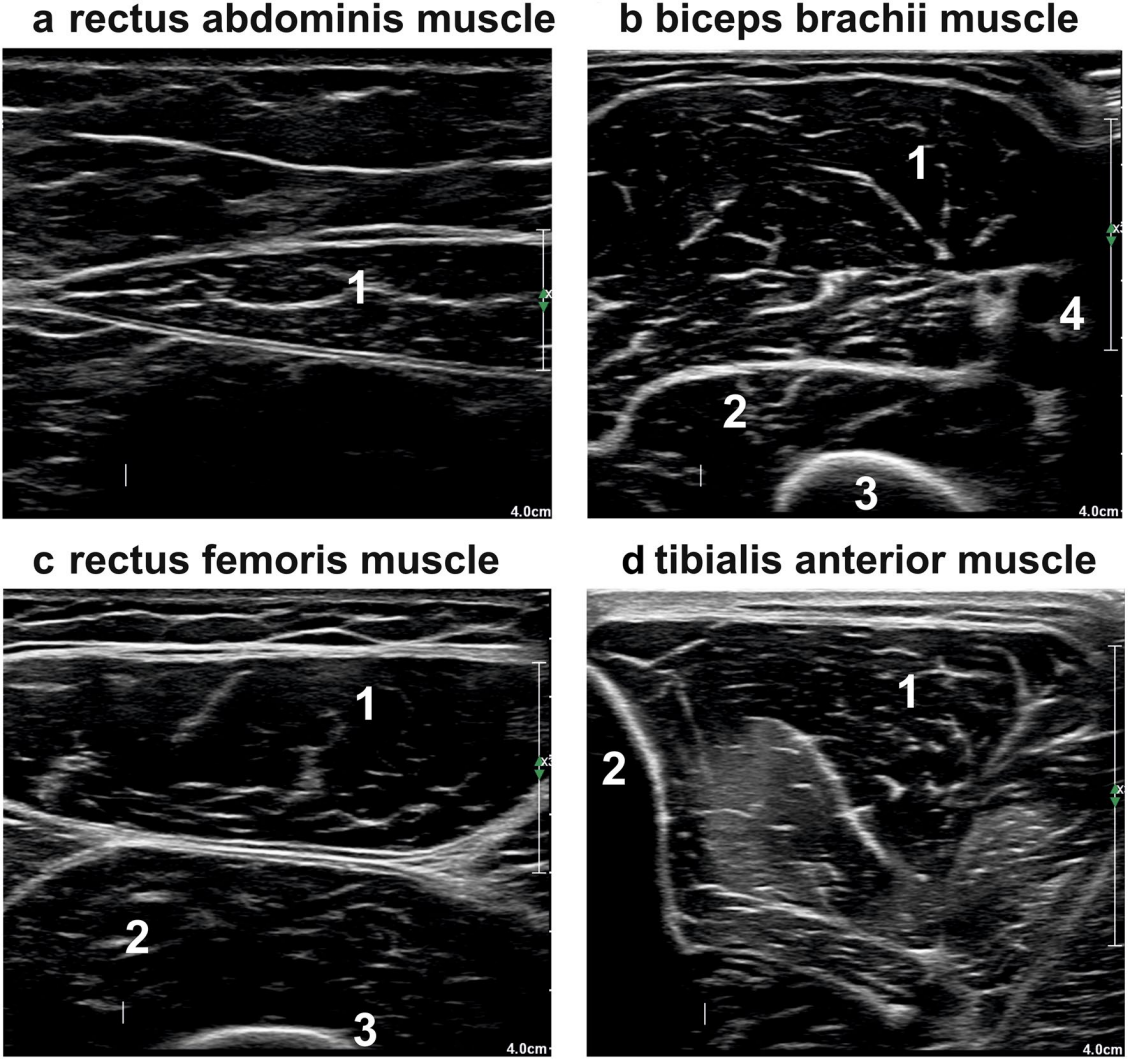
Depicts examples of sectional sonographic images of muscles surrounded by the typical anatomic landmarks a: rectus abdominis muscle (1: rectus abdominis muscle); b: biceps brachii muscle (1: biceps brachii muscle; 2: brachial muscle; 3: humerus; 4: neurovascular bundle); c: rectus femoris muscle (1: rectus femoris; 2: vastus intermedius muscle; 3: femur); d: tibialis anterior muscle: (1: tibialis anterior muscle; 2: tibia).
